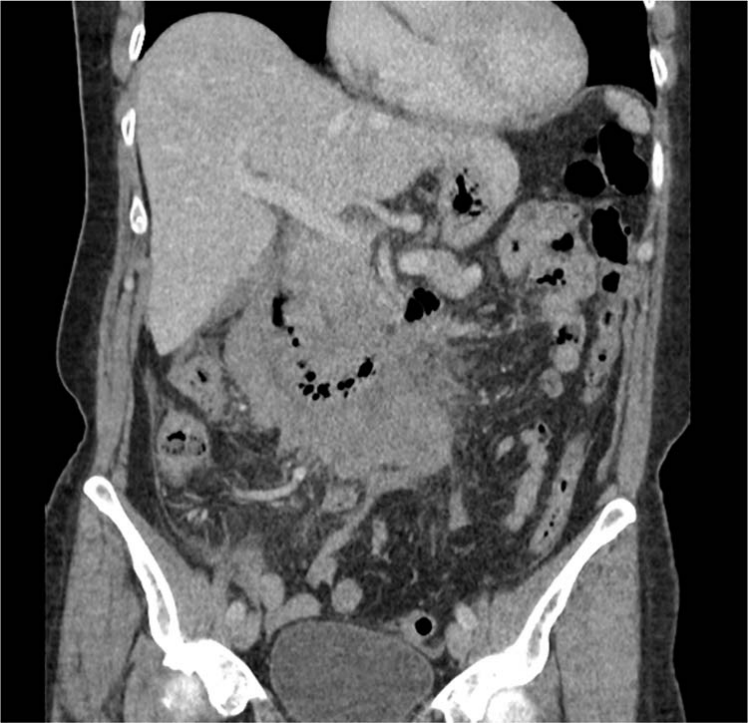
CT abdomen, coronal view. Hyperdense fluid centered around the duodenum and tracking into the paracolic gutters. Appearance concerning for paraduodenal hemorrhage, infiltrating mass was considered less likely.
